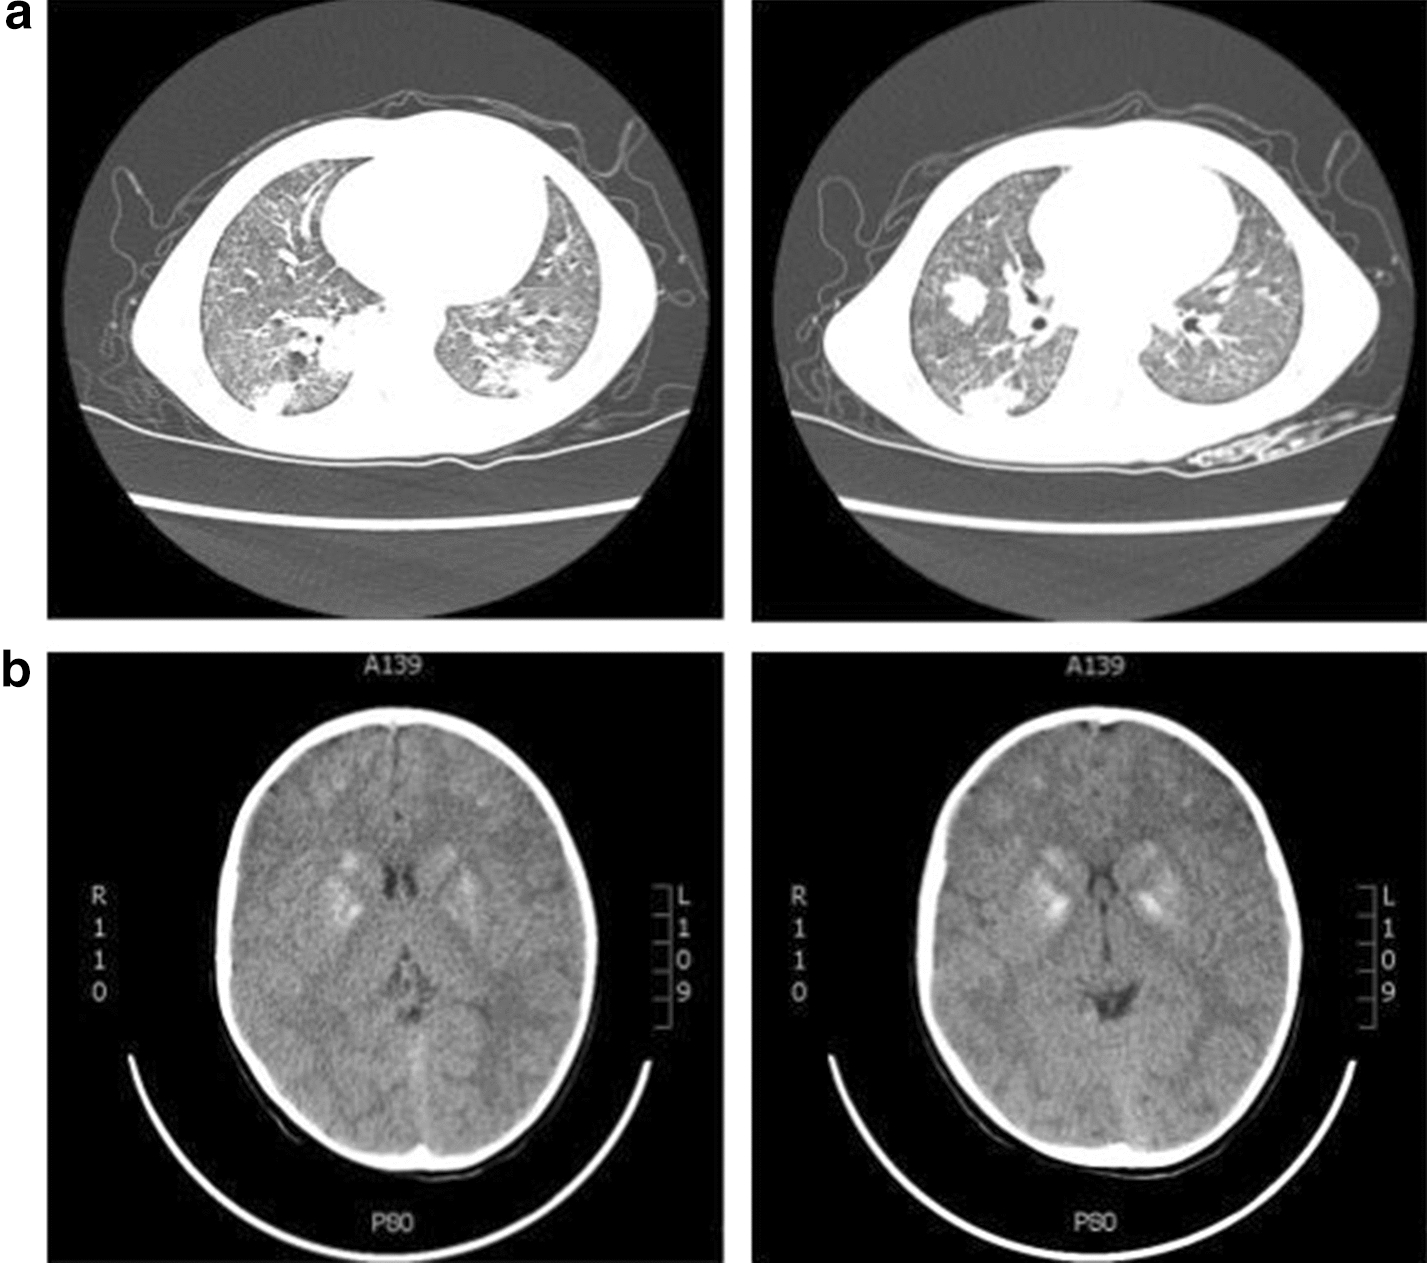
a Chest CT scan. Bilateral focal areas of parenchymal consolidations, some of them with air bronchograms and with nodular appearance, distributed in the peripheral portions of the lower zones. Bilateral widespread septal thickening and peripheral tree in-bud changes in both lungs is observed too. Lobar air-trapping (ILL) on expiration was present. b Brain CT scan. Calcifications at the level of the caudate nuclei, lenticular nuclei and subcortical region of both frontal lobes are shown in the images
